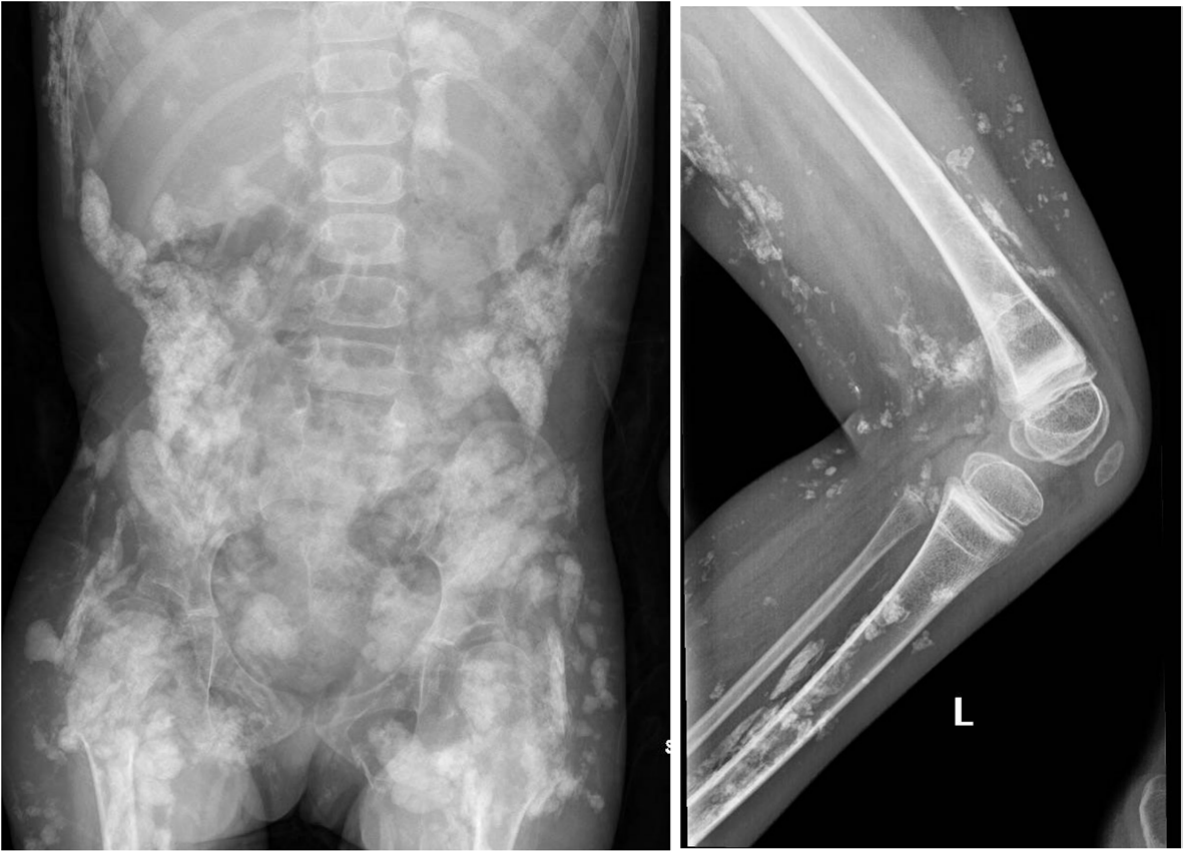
X-ray of abdomen and left knee with extensive amorphous and multilobulated calcinosis in a 7-year-old female with refractory JDM
